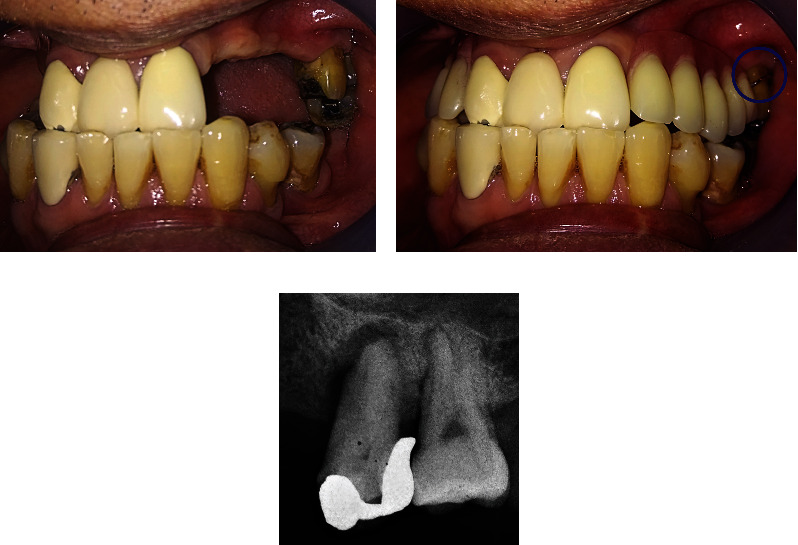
Initial condition. (a) Intraoral condition of gingival region tooth #26 showed recession without visible abscess. (b) Intraoral condition when the patient wore denture with a single clasp on tooth #26 (shown in blue circle). (c) Initial radiograph condition. Overhanging restoration on the distal part of the crown. Radiolucency surrounded the mesial and apical parts of the roots.
